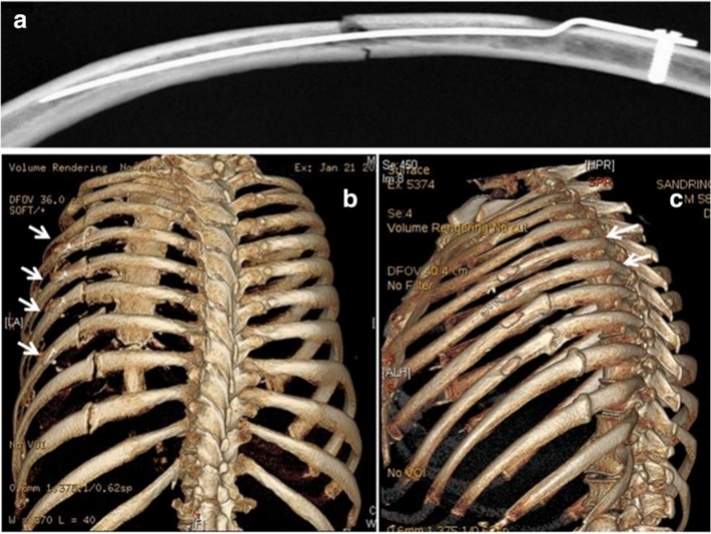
a. Intramedullary splint in situ [10, 11]. b. Splints used to fix fractures in left ribs 5–8. 3D CT scan at 3 months shows good alignment but little bony bridging across the fracture site (except for the fifth rib – most superior rib fixed). In fact the amount of bony callus formation is comparable to the 9^th^ and 10^th^ rib fracture sites which were treated conservatively. c. Same patient at 6 months with complete healing of all ribs. The fracture sites in ribs 5 and 6 are imperceptible
